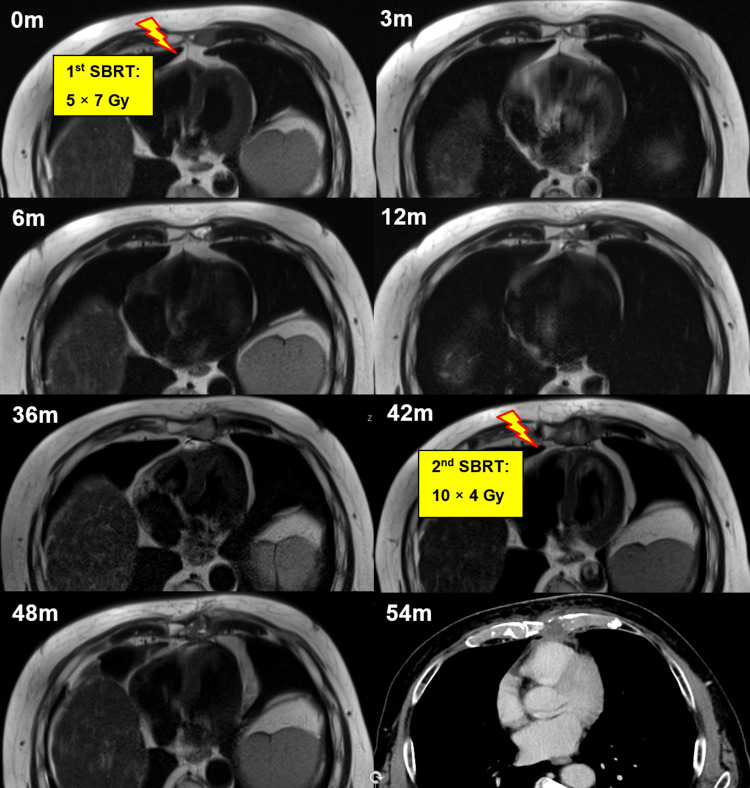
T2-weighted MR images showing the sternal metastasis over time. At baseline (0m) the first SBRT with 5 × 7 Gy was delivered using the CyberKnife (Accuray Inc., Sunnyvale, CA). Follow-up scans were performed at three, six, 12, and 36 months (3m, 6m, 12m, 36m). After local progression was confirmed at 42 months (42m), re-SBRT with 10 × 4 Gy was delivered with the CyberKnife. MRI and CT images confirmed that the lesion remained controlled at six and 12 months after re-SBRT (48m, 54m). The patient died 14 months after re-SBRT due to multifocal progression. SBRT = stereotactic body radiotherapy
